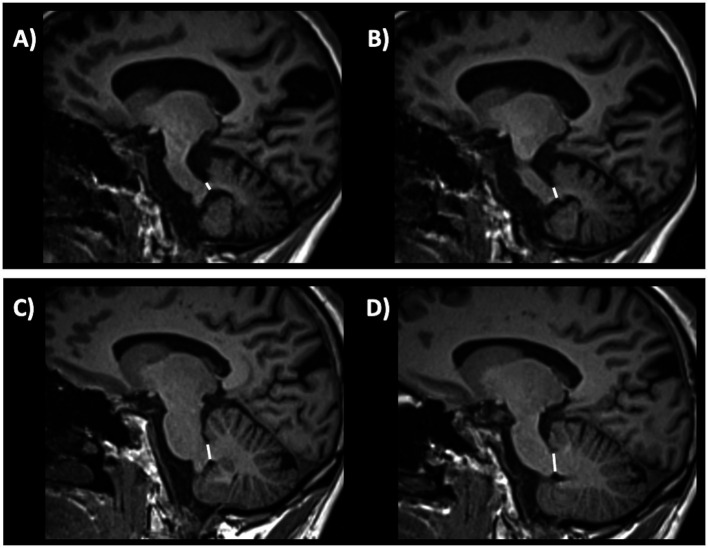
Three-tesla brain T1-weighted parasagittal MR images showing how to measure the middle cerebellar peduncle width measurements, in a patient with multiple system atrophy (A,B) and in a patient with Parkinson’s disease (C,D). This structure is more atrophic in multiple system atrophy than in Parkinson’s disease.
